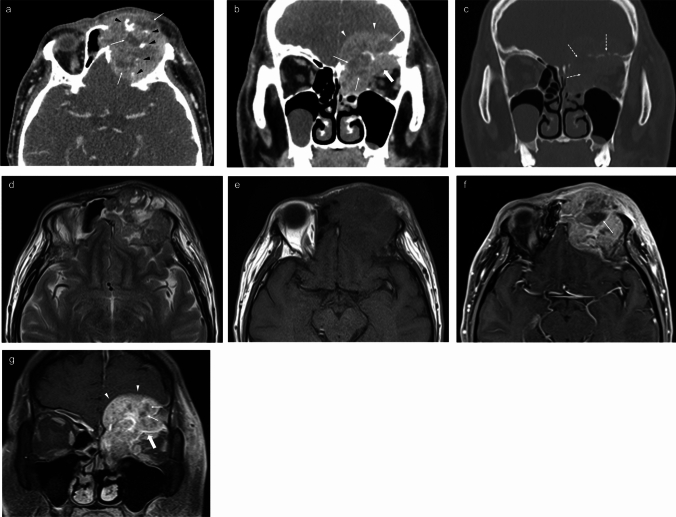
Sinonasal nuclear protein in testis carcinoma (case 3). Contrast-enhanced CT axial image (a) and coronal image (b) show a tumor in the left ethmoid sinus with heterogeneous moderate enhancement, with a necrotic area inside (white arrow) and irregular calcification (black arrowheads). The lesion demonstrates intracranial extension via destruction of the skull base and left supraorbital wall (arrowheads) and intraorbital extension via destruction of the medial lateral orbital wall (bold arrows). Bone algorithm CT coronal image (c) shows absence of the skull base and medial orbital wall, indicating bone destruction (dotted arrows). The solid component of the lesion shows high signal intensity in the T2-weighted image (d) and isointense signal on T1-weighted image (e). Post-contrast T1 axial (f) and coronal (g) images show heterogeneous moderate enhancement of the tumor, with internal necrotic areas (arrows), intracranial extension (arrowheads) and intraorbital extension (bold arrows)
